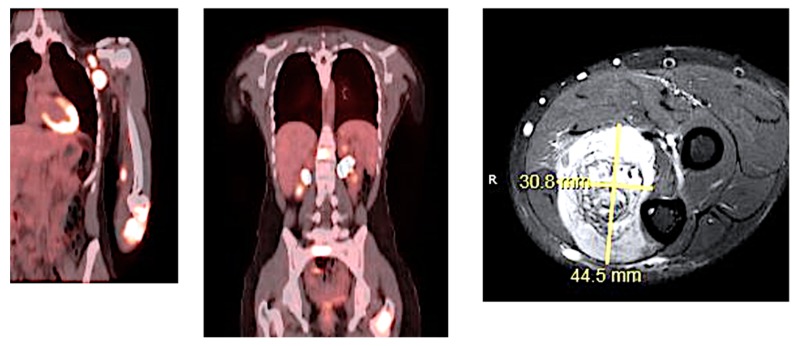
PET/CT and MRI of 19 y/o patient with Stage IV extremity alveolar RMS. Uptake can be appreciated in the left axillary node, left elbow and left femoral head.
